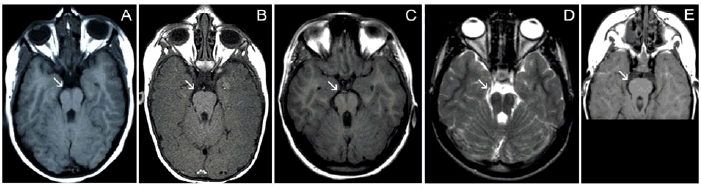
Magnetic resonance imaging (MRI) showing characteristic molar tooth sign (MTS) in five patients with Joubert syndrome/cerebello-oculo-renal syndrome due to mutations in *CEP290* (all are axial sections through midbrain). Images organized from left to right; arrows indicate MTS of midbrain present in all due to midbrain malformation with hypoplastic cerebellar vermis and midline cleft (all images are T1 weighted except for panel D which is T2 weighted). A) CORS-1 at age 5 years; B) LCA-JS-1 at age 7 years; C) LCA-JS-2 II-1 at age 14 years; D) LCA-JS-2 II-2 at age 17 years and E) LCA-JS-3 at age 2 years.
